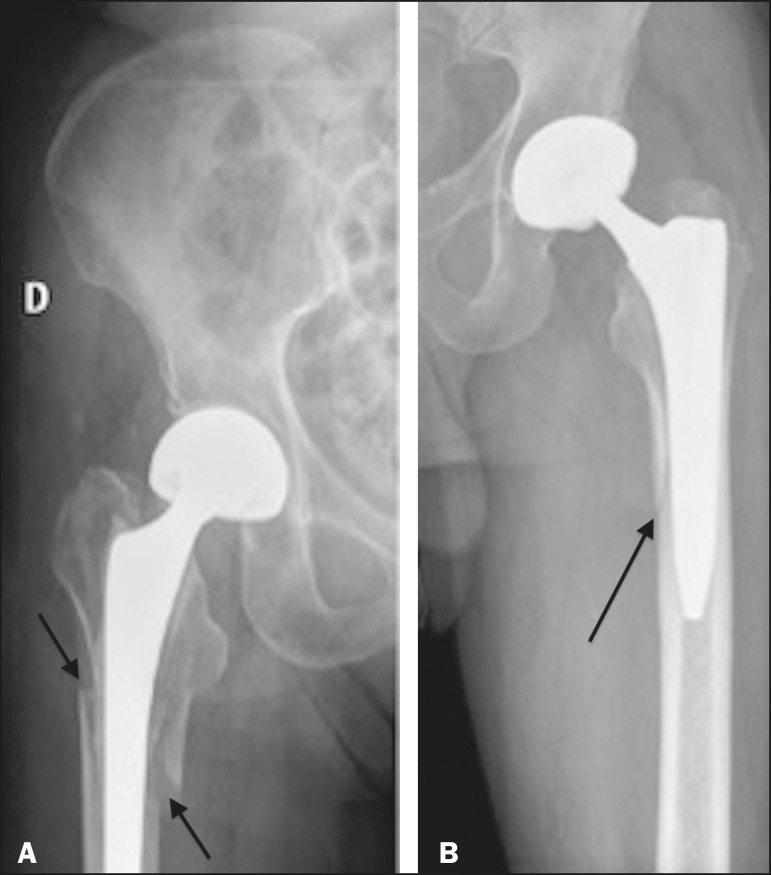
A: Anteroposterior X-ray of the right hip showing periprosthetic fracture (arrows) in the femoral component (Vancouver subtype B1). B: Anteroposterior X-ray of the left hip showing cortical fracture (arrow) at the medial margin of the femoral prosthetic component (Vancouver subtype B1).
